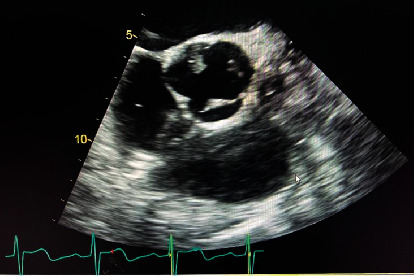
Echocardiogram demonstrating moderate-size aortic valve vegetations in each tricuspid valve leaflet.
